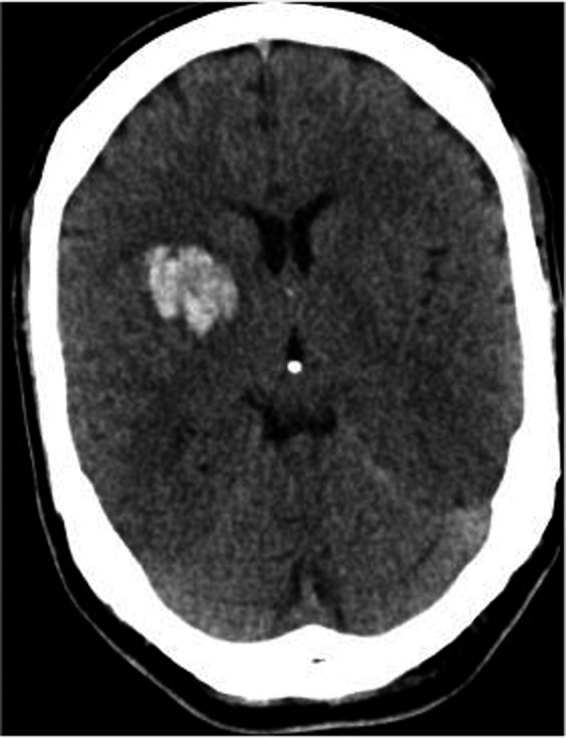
Brain CT scan showed a SICH by a 45‐year‐old patient, firstly diagnosed with blood hypertension. The patient was presented with a weakness on the left side and hypertensive crisis
